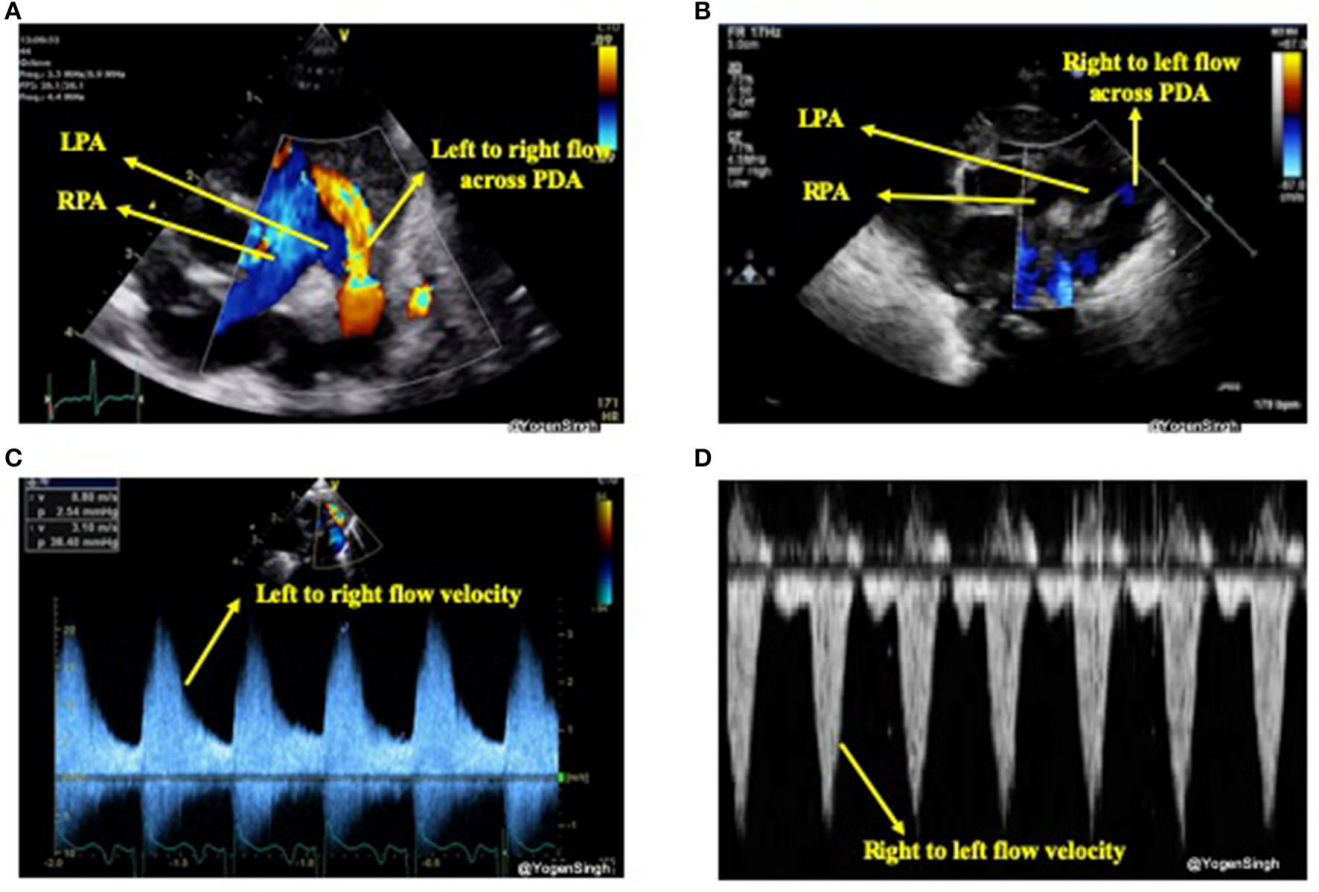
Assessment of PDA shunt direction on color flow and with Doppler application. (A) Left to right shunt seen as red (blood coming toward probe) while blood in branch pulmonary arteries seen as blue (blood going away from probe); (B) showing right to left shunt seen as blue color—similar to branch pulmonary arteries in a view “three legged trouser;” (C) Doppler assessment showing left to right shunt (above the baseline as blood coming toward the probe); and (D) Doppler assessment showing right to left shunt (below the baseline as blood going away from the probe). LPA, left pulmonary artery; RPA, right pulmonary artery; PDA, patent ductus arteriosus.
